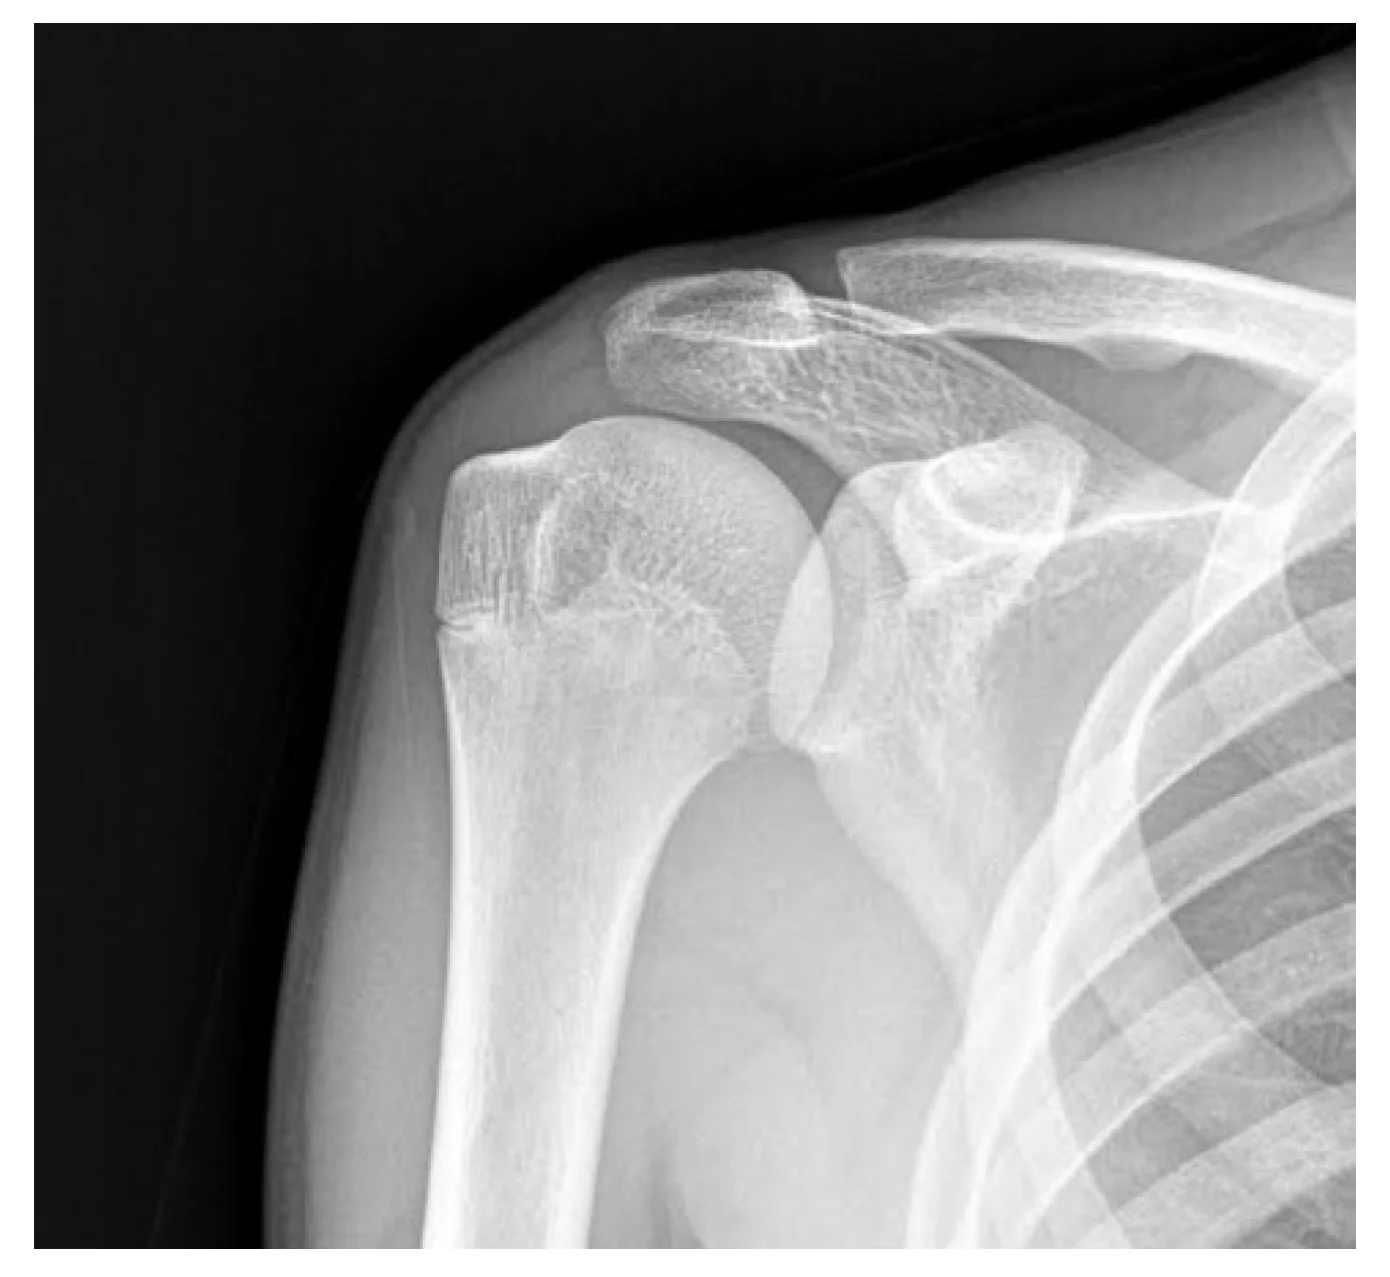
Plain radiograph of the right shoulder. An anteroposterior radiograph showed no osseous abnormality, fracture, dislocation or malalignment.
